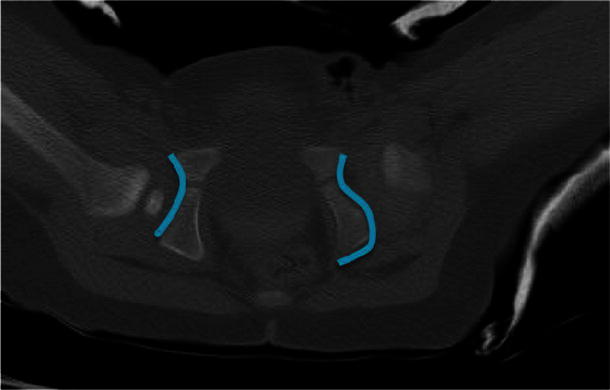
Postreduction single slice CT image showing the morphology of dysplasia
